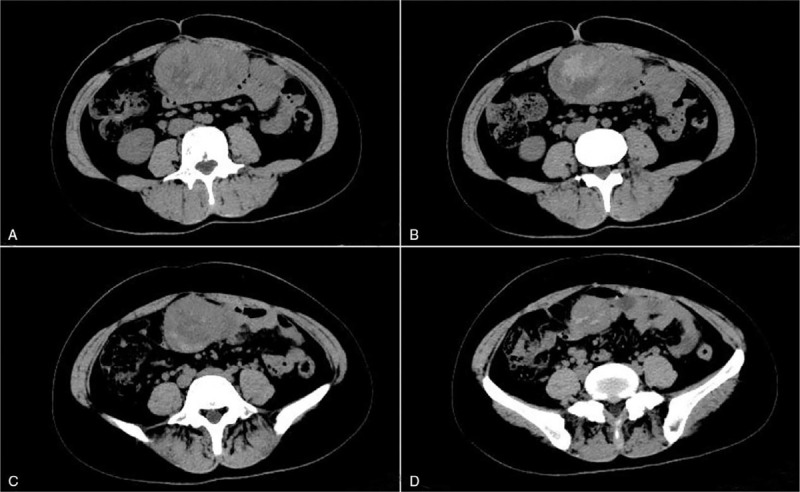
Abdominal computed tomography of case 1. (A and B) A 5.8 cm × 9.0 cm mass was found at the umbilicus level. (C and D) A 3.5 cm × 4.5 cm soft tissue mass was observed at 5 cm below the umbilicus and at the outer edge of the left rectus abdominis.
